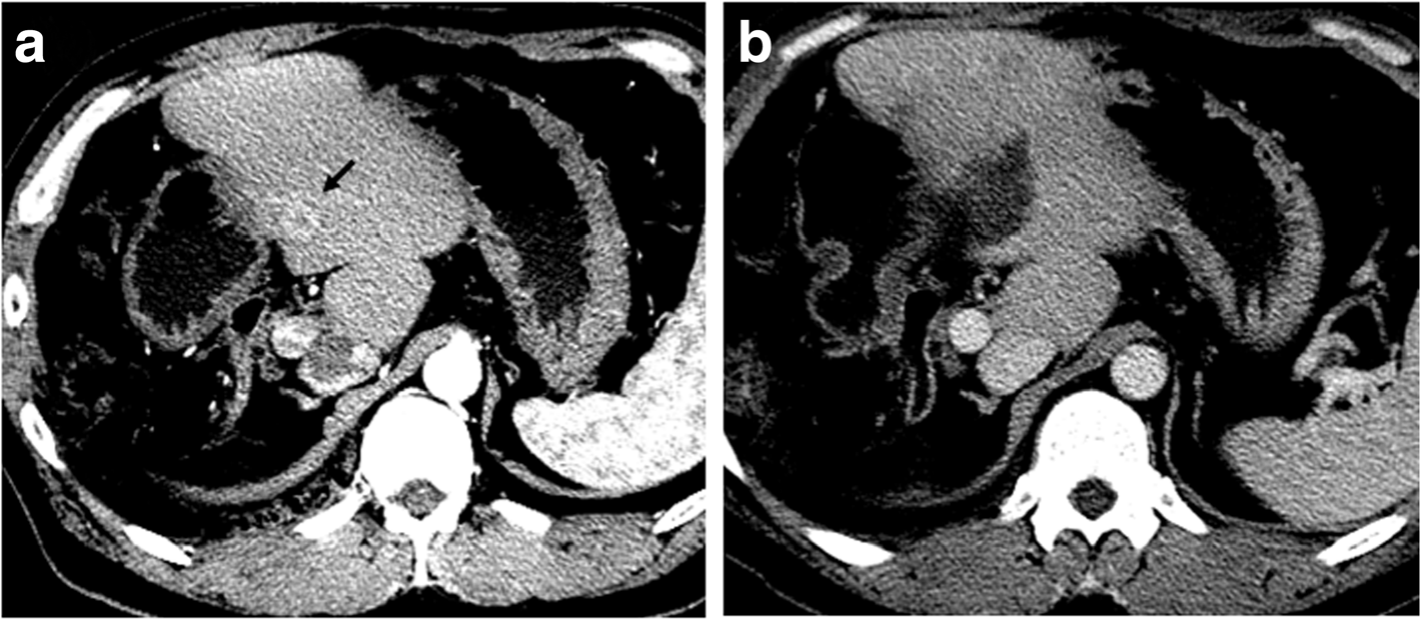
Computed tomography images of a recurrent lesion treated with radio frequency ablation. a A peripherally well-enhanced tumor is seen in segment 3 (*arrow*). b The index tumor is completely ablated with radio frequency ablation
